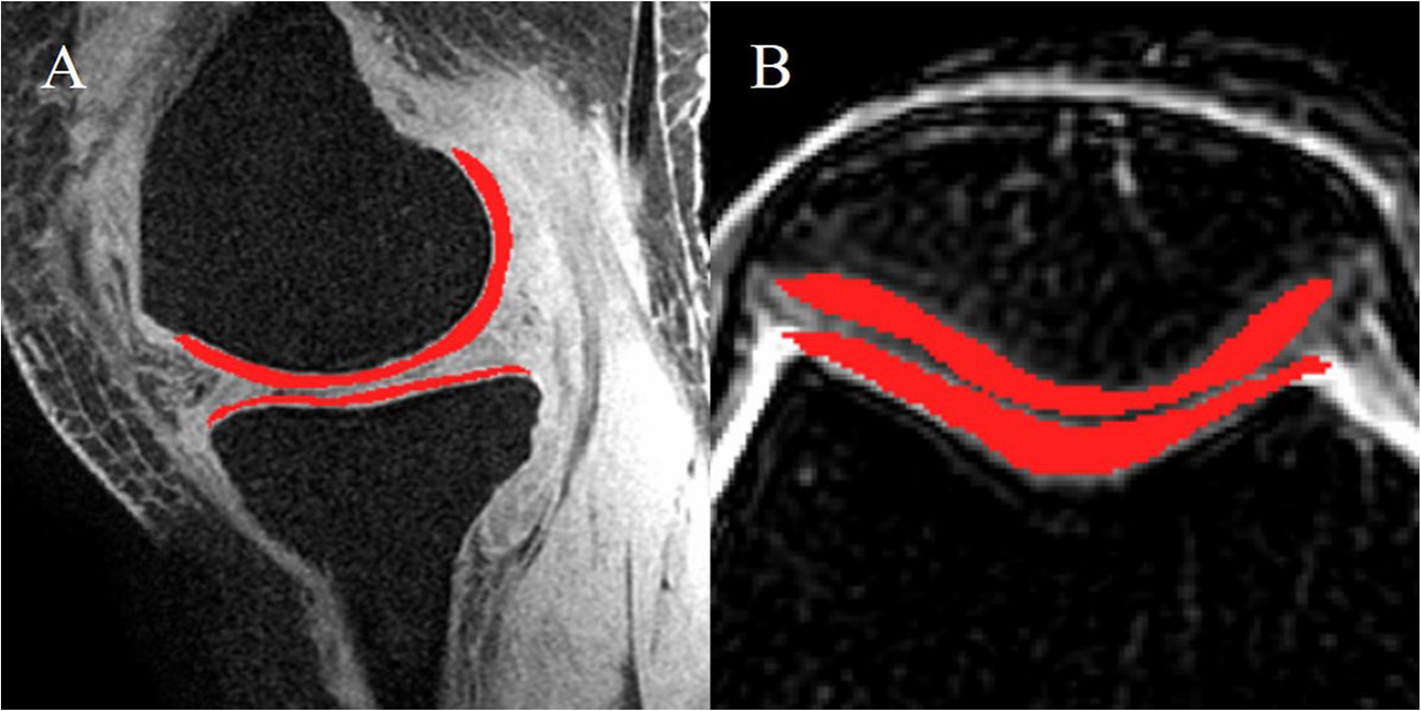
The manually traced ROIs contain the cartilage on the 3D fat-suppressed SPGR for measuring cartilage volume (a) and transverse fat-suppressed T2-weighted images for quantitative MRI measures (b)
